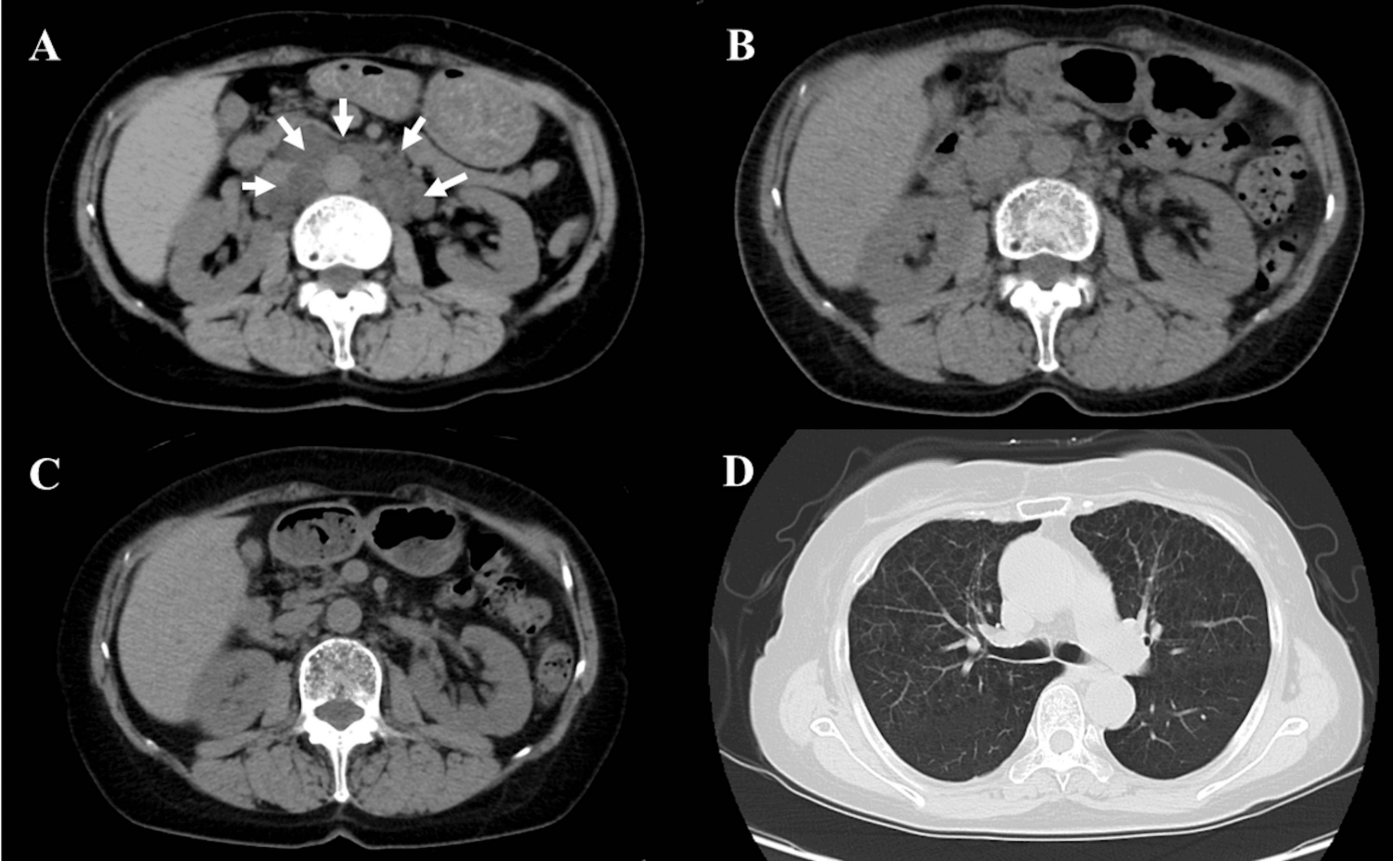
Abdominal and pulmonary CT images over time after presentation to our hospital. CT images at the initial visit (A) showed an increase and enlargement of multiple abdominal nodules (arrows). A CT image taken two months after starting medication (B) revealed a reduction in the size of the abdominal nodules. After nine months of medication (C), the abdominal nodules had nearly disappeared. CT imaging of the pulmonary lesions (D) showed no significant changes throughout the disease course.
